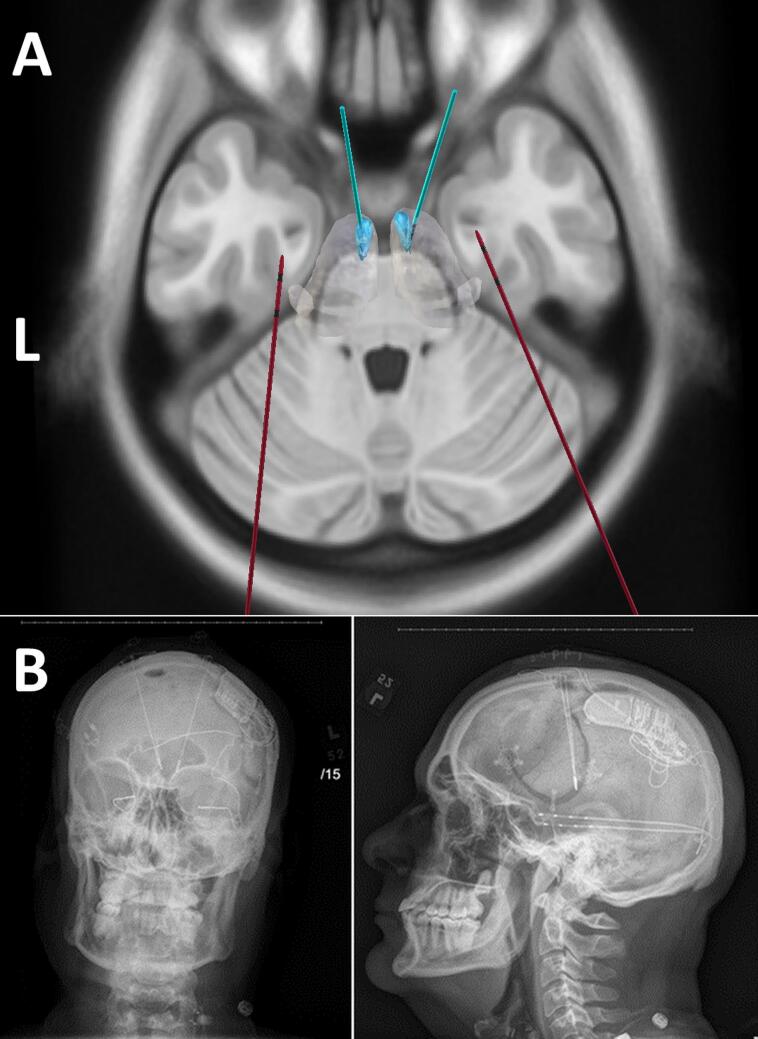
(A) Multimodal neuromodulation implant: An example of a patient who had received both anterior nucleus (ANT) of thalamus deep brain stimulation (DBS) as well as hippocampal responsive neurostimulation (RNS) and the vagal nerve stimulator (VNS): The accuracy of implantation was determined by co-registering post-implant CT into pre-operative MRI images using non-linear transform in advanced normalization toolbox. Thalamus Optimized Multi Atlas Segmentation (THOMAS) segmentation algorithm was used to generate patient specific thalamic nucleic segmentation (blue regions represent the bilateral ANT nuclei, the gray blob represents the thalamic boundary). DBS electrodes (cyan) were localized to the ANT and the bilateral RNS electrodes (red) were localized to the hippocampi. (B) Anteroposterior and lateral fluoroscopy images showing the neurostimulator placements for vagus nerve stimulation (VNS), responsive neurostimulation (RNS), and deep brain stimulation (DBS). VNS lead with terminal electrodes placed adjacent to a segment of the left vagus nerve (cuff on vagus nerve). (For interpretation of the references to colour in this figure legend, the reader is referred to the web version of this article.)
